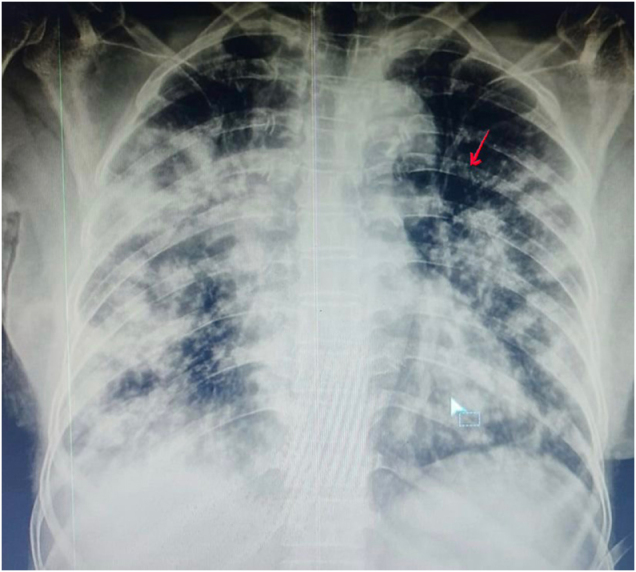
Chest radiograph showing diffuse bilateral nodular densities with cavitation (red arrows).
